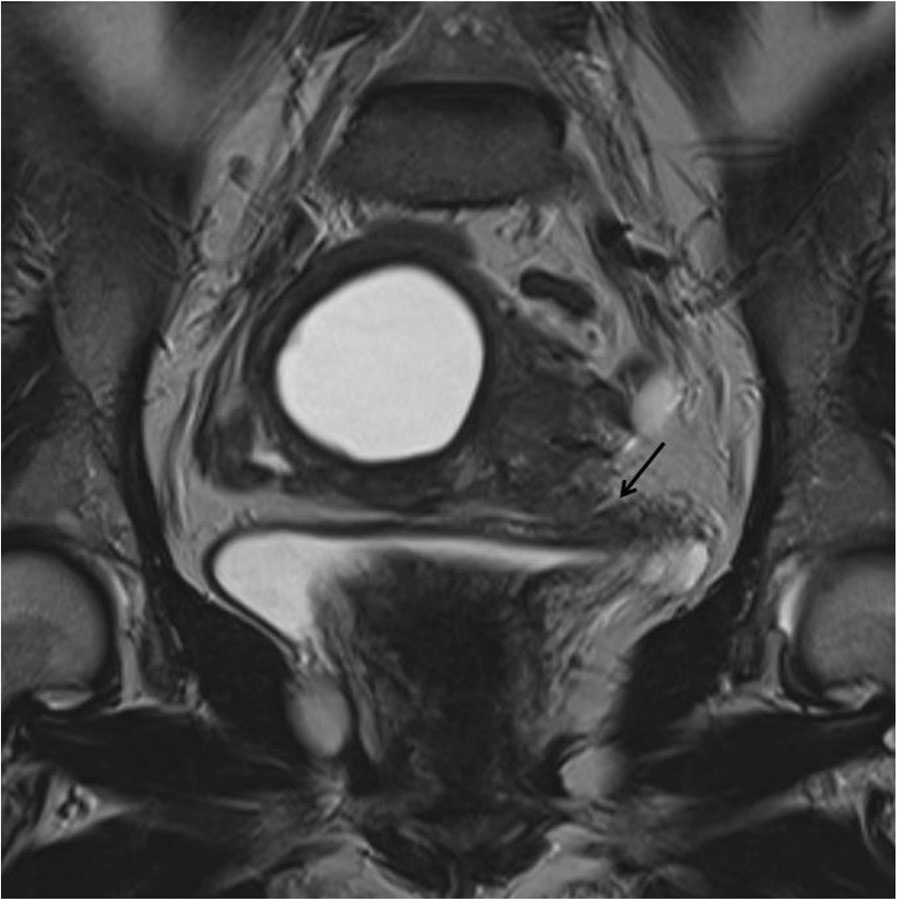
A 57-year-old woman with EC stage IVA. Coronal T2-weighted MR image showing a slightly hypointense tissue extending from the left parametrium to focally disrupt the urinary bladder wall on the left side (arrow)
